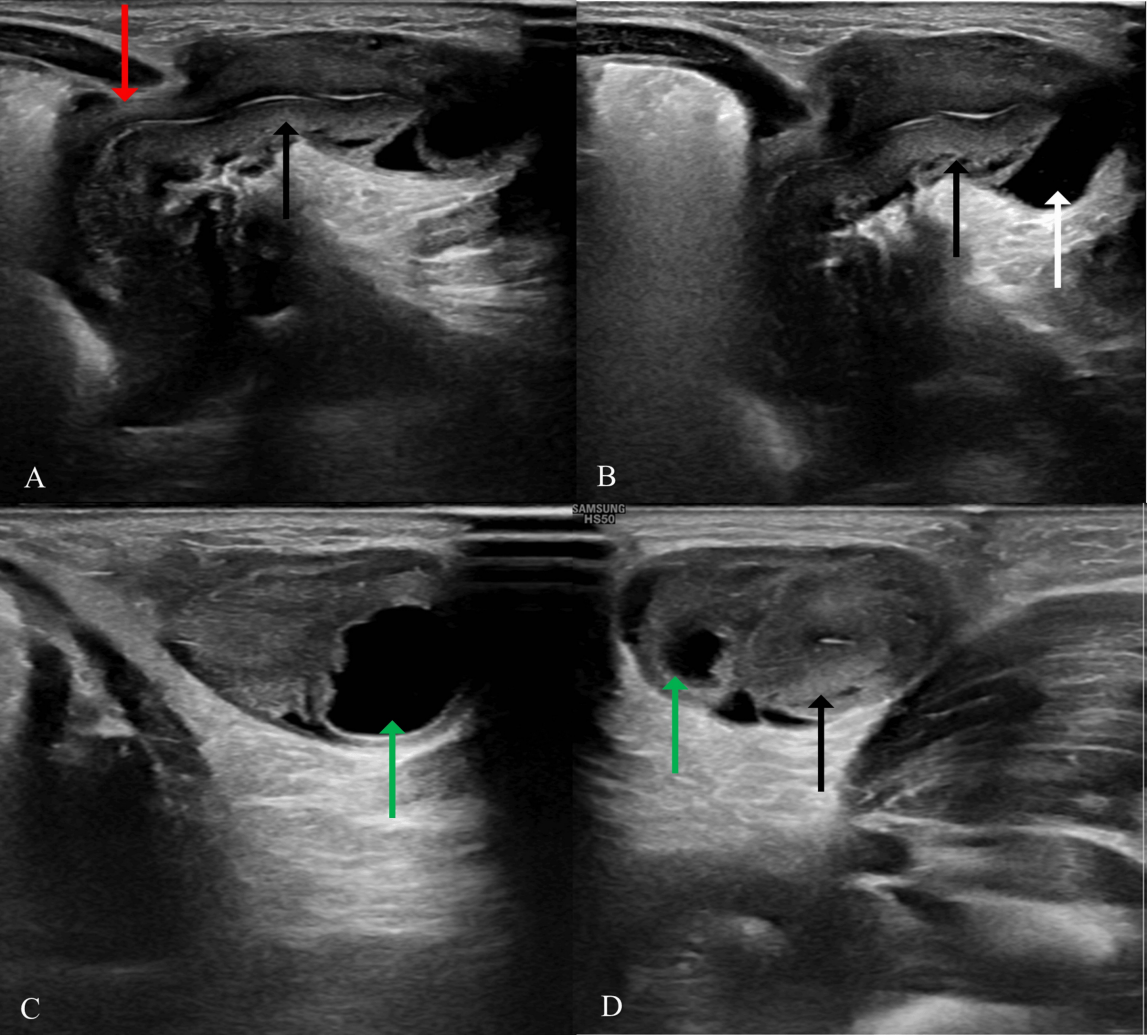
Ultrasonography images of the left inguinal region (A)-(B) Sagittal images show the uterus (black arrows), mild fluid (white arrow) and defect of the hernia (red arrow); (C)-(D) Axial images show the uterus (black arrows) and ovary (green arrow)
